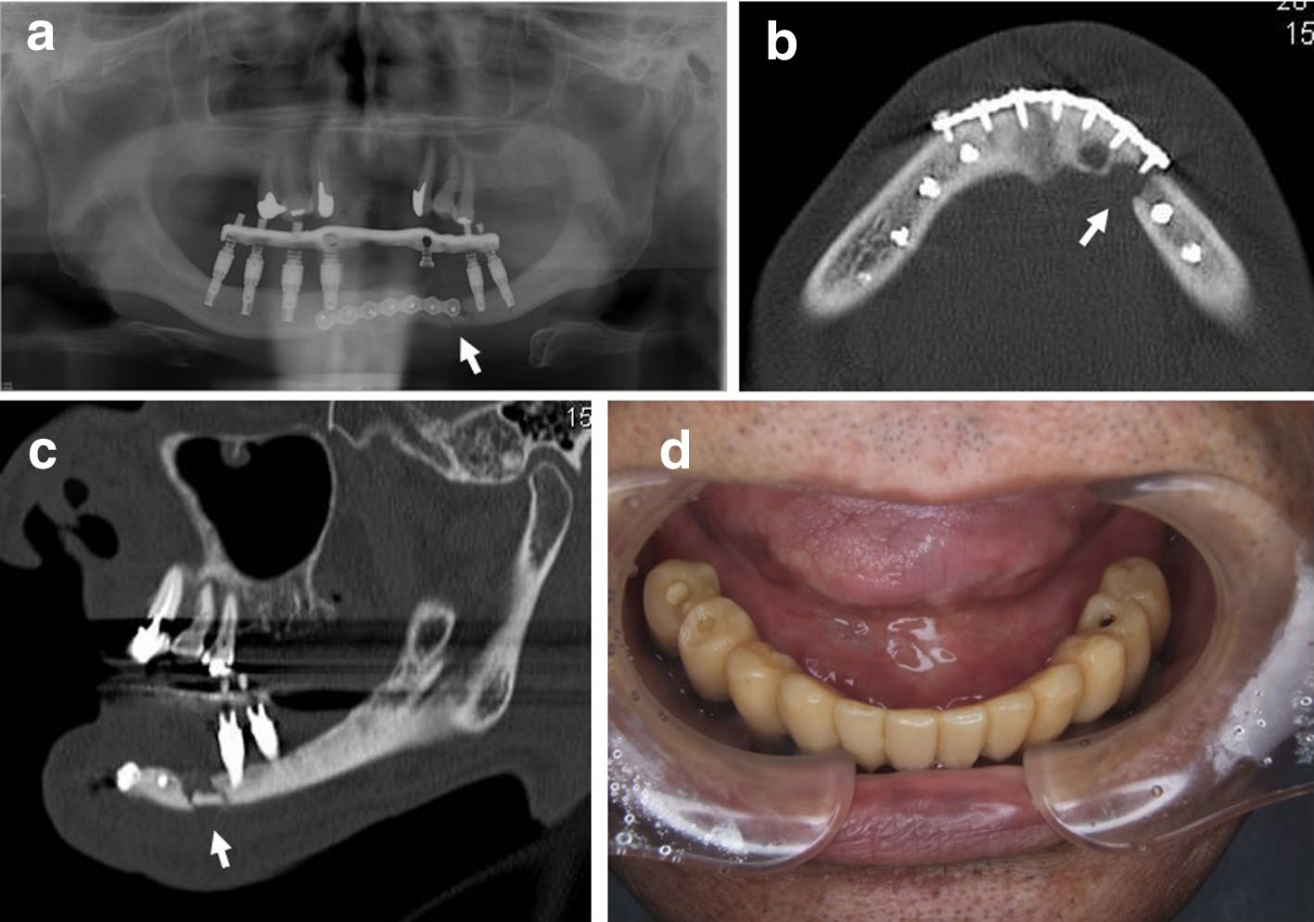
a Fracture ( →) in the left mandibular premolar on panoramic radiograph, b fracture ( →) on horizontal CT image, c the fracture site ( →) had the smallest bone height diameter on sagittal CT image and d intraoral photograph after the seating of superstructure
